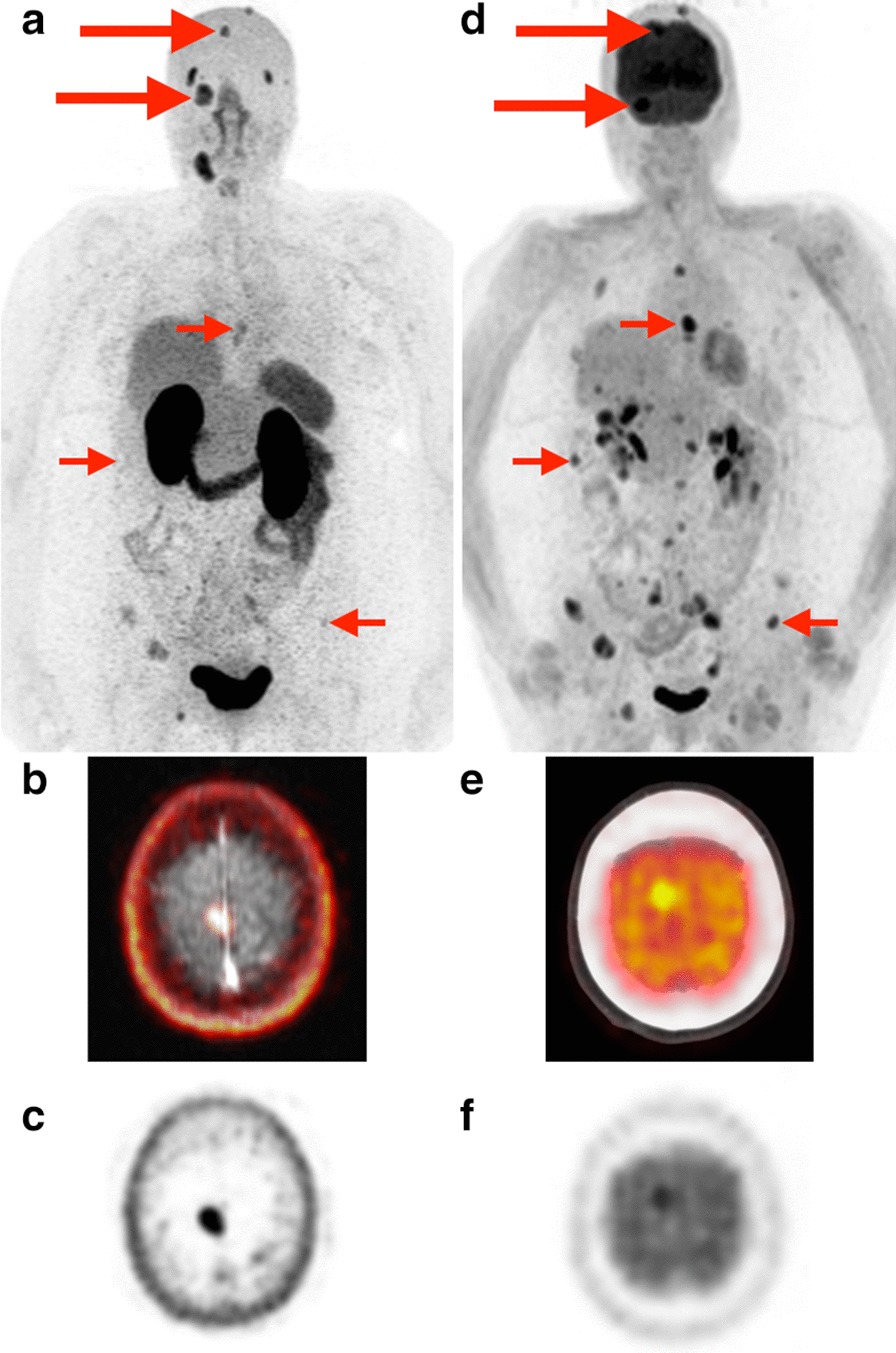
Dural and skull base metastases.[^68^Ga]Ga-PSMA-11 PET MIP (a) and 2-[^18^F]FDG PET MIP (d) in a patient (Patient 2) with Hurthle cell carcinoma. Dural and skull base metastases (large arrows) are better delineated on PSMA PET due to lack of background brain uptake (example: right falcine dural metastasis better seen on fused axial PSMA PET/MRI (b) and axial PSMA PET (c) than on fused axial FDG PET/CT (e) and axial FDG PET (f). Extensive metastatic disease involving the left adrenal gland, ribs, thoracolumbar spine, and pelvis is better seen on FDG PET in this patient
